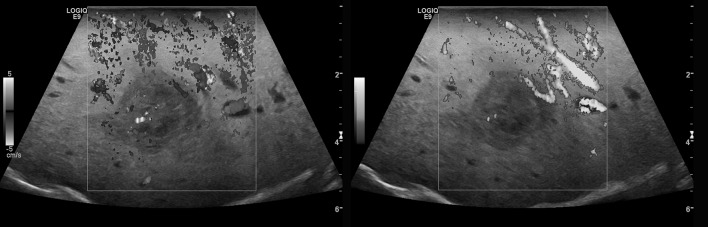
CCDS (left) and Power Doppler (right) for characterization of the vascularization of an HCC lesion.
